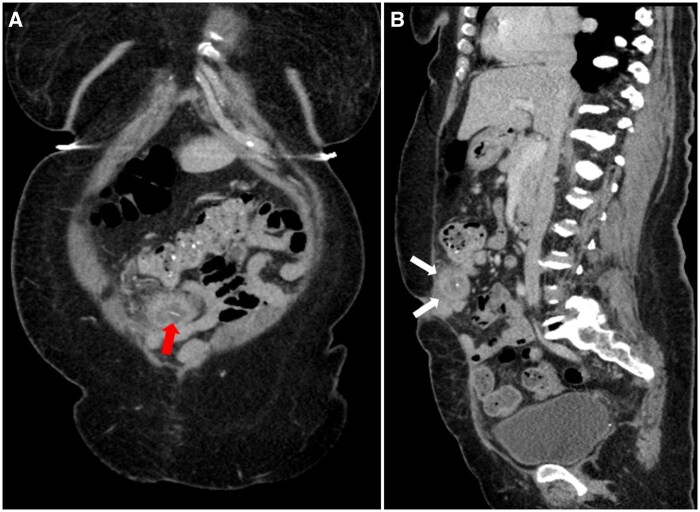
Case 3. Coronal (A) and sagittal (B) CT with PV contrast showing the retro-umbilical inflammatory mass (white arrows) and a linear hyperdense fish bone within the mass (red arrow).
